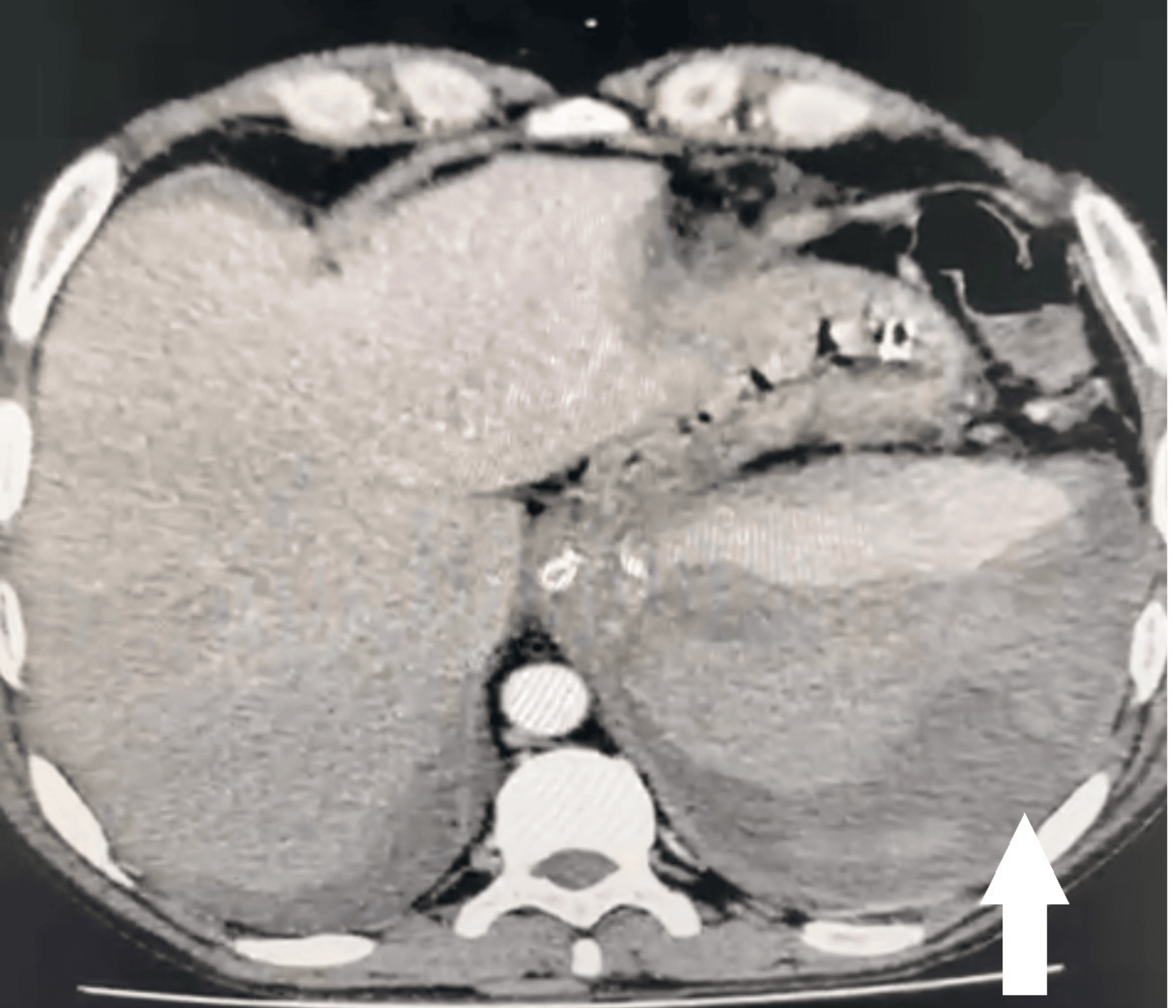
Intrasplenic pseudocyst rupture in chronic pancreatitis Axial CT showing subcapsular splenic hematoma with rupture (white arrow).
